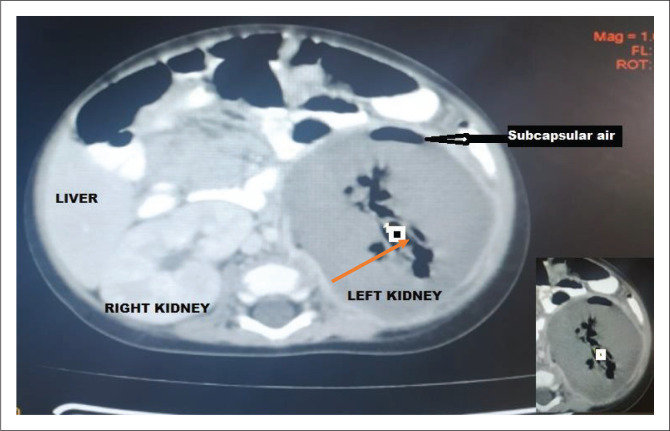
Contrast enhanced computed tomogram of an emphysematous pyelonephritis patient at the Usmanu Danfodiyo University Teaching Hospital, Sokoto, Nigeria, November 2019. Figure shows left renomegaly, contrast enhancement and multiple air densities within the calyceal system (orange arrow).
